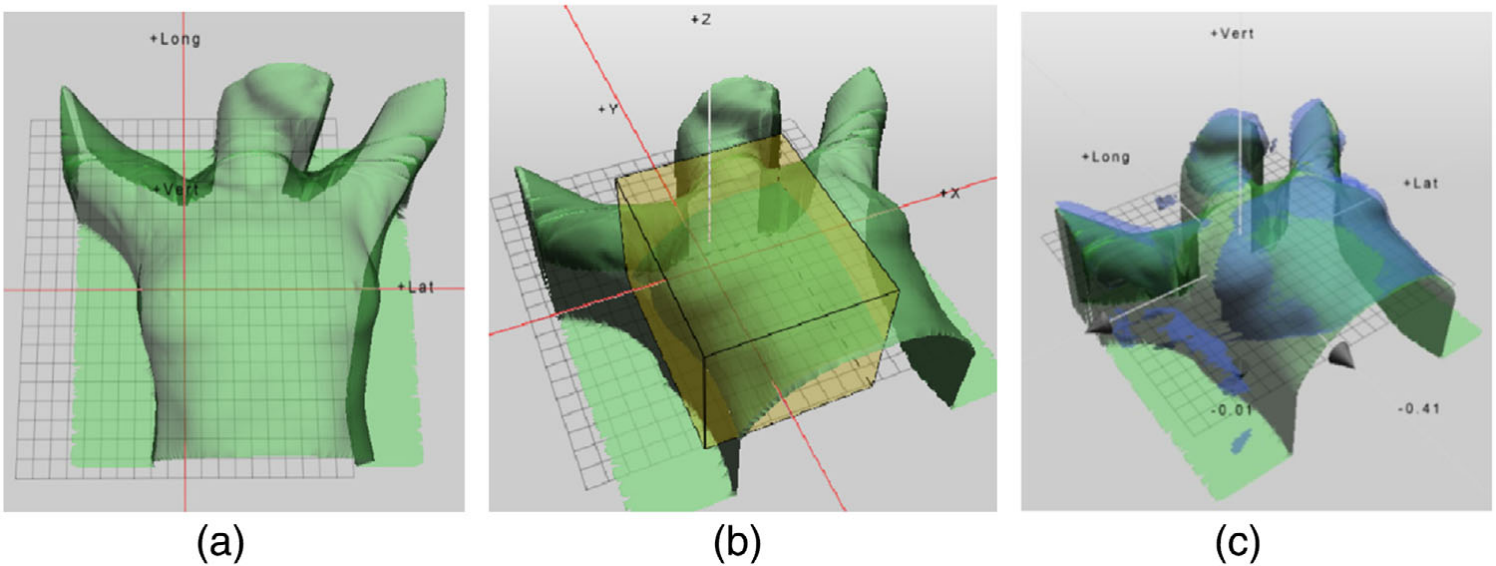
(a) Extracted from the planning CT system was used as Sentinel reference CTref. (b) Optical scan area range from the supraclavicularregion to submammary sulcus. (c) Images of surface registration: green is the reference contour surface, and blue is new contour surface obtained by laser scan
